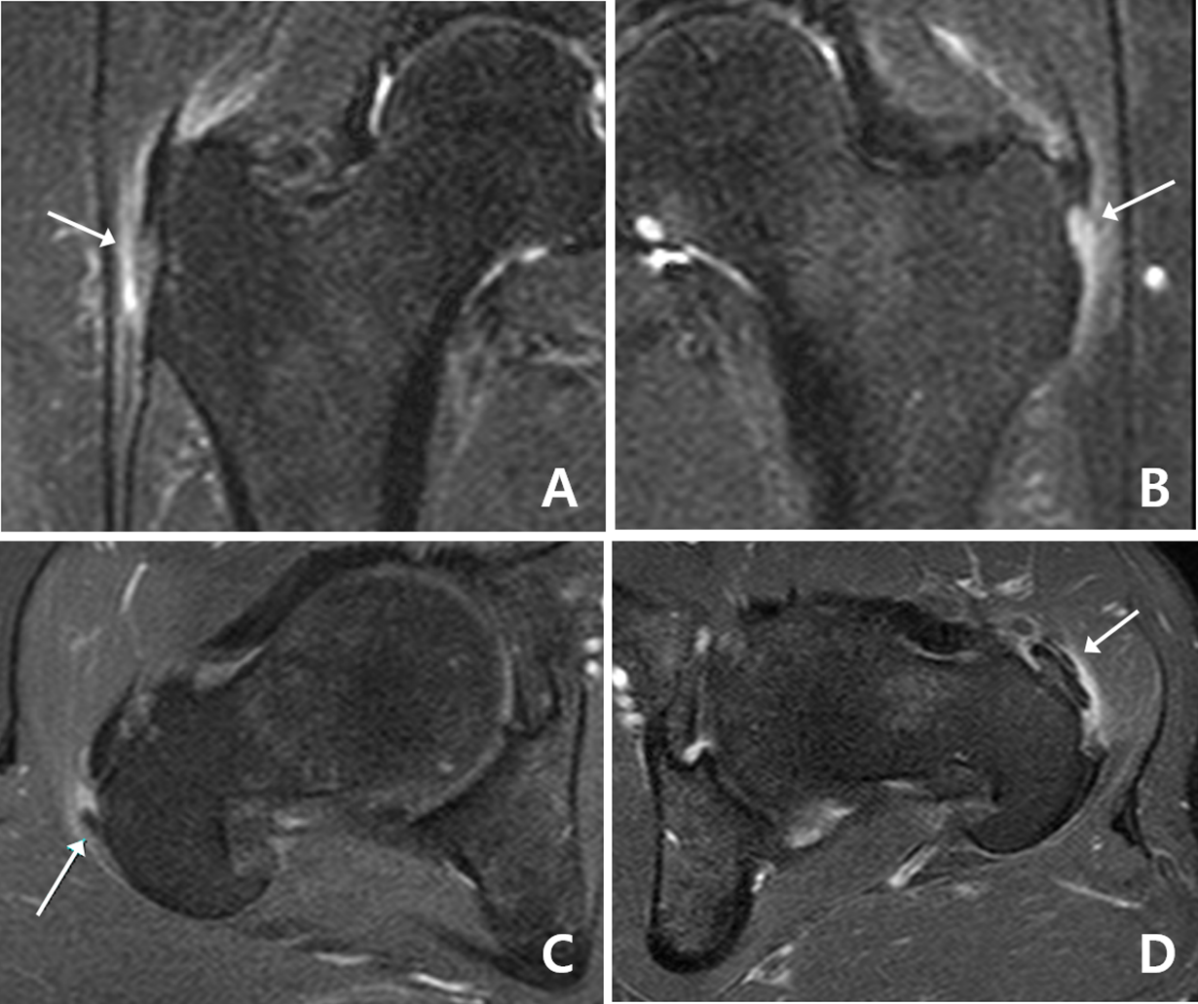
Findings of magnetic resonance imaging of gluteal tendinopathy. A: Mild increased intrasubstance signal and surrounding edema (arrow) suggests gluteus medius tendinosis with peritendinitis. B: Marked increased intrasubstance signal and ill-definition of the gluteus medius tendon near the insertion on the lateral facet (arrow) suggests insertional partial tear. C: Contrast-enhanced axial T1 with fat saturation MR images demonstrates a hypointense calcium deposit (arrow) in the gluteus medius tendon with surrounding edema. D: Mild increased intrasubstance signal and surrounding edema (arrow) suggests gluteus minimus tendinosis with peritendinitis.
